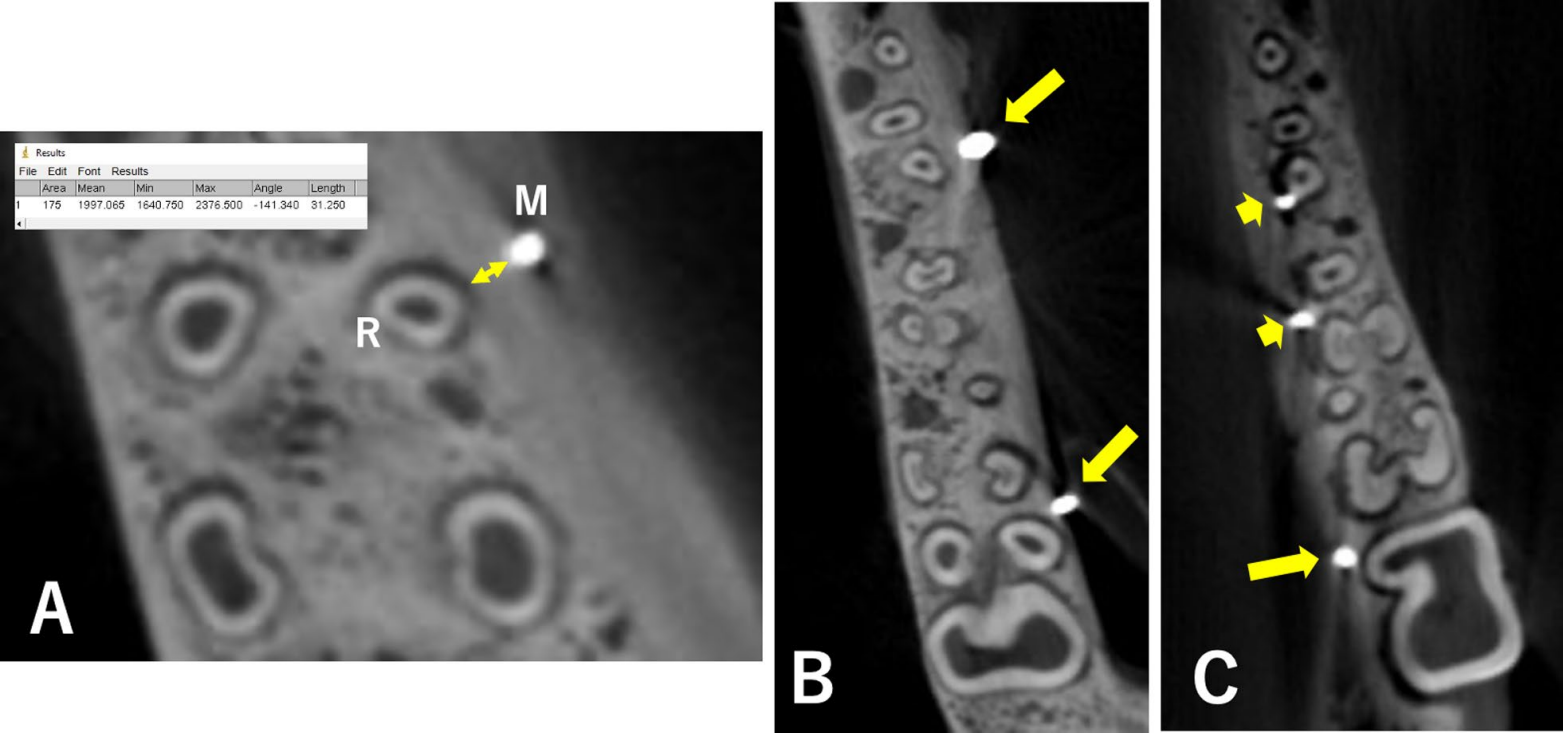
Cross-sectional cone beam computed tomography (CBCT) image showing how the distance (A: yellow arrow line) between the miniscrew (M) and the root (R) was measured. CBCT image showing some miniscrews were some distance from the roots (B, C: arrow), and some were close to the roots (C: arrowhead)
